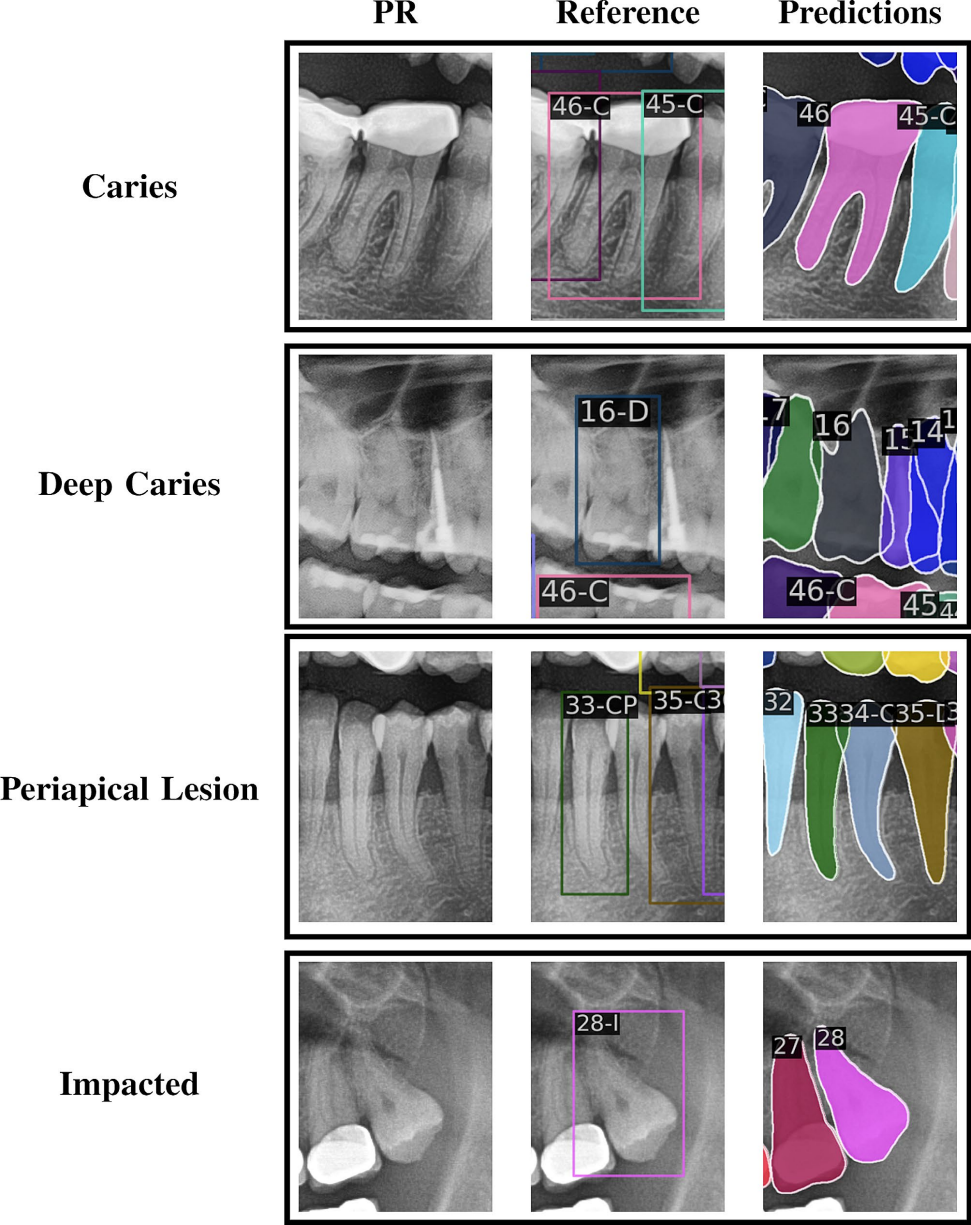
Misdiagnosed DENTEX annotations. A misdiagnosis (false positive) is shown for each diagnosis, which are selected based on the maximum difference between a diagnosis probability and whether that diagnosis is annotated. Note that the DENTEX dataset only annotates diagnosed teeth, whereas the current method predicts all teeth. The label is the tooth’s FDI number with C = early caries, D = deep caries, P = periapical lesion, I = impacted
